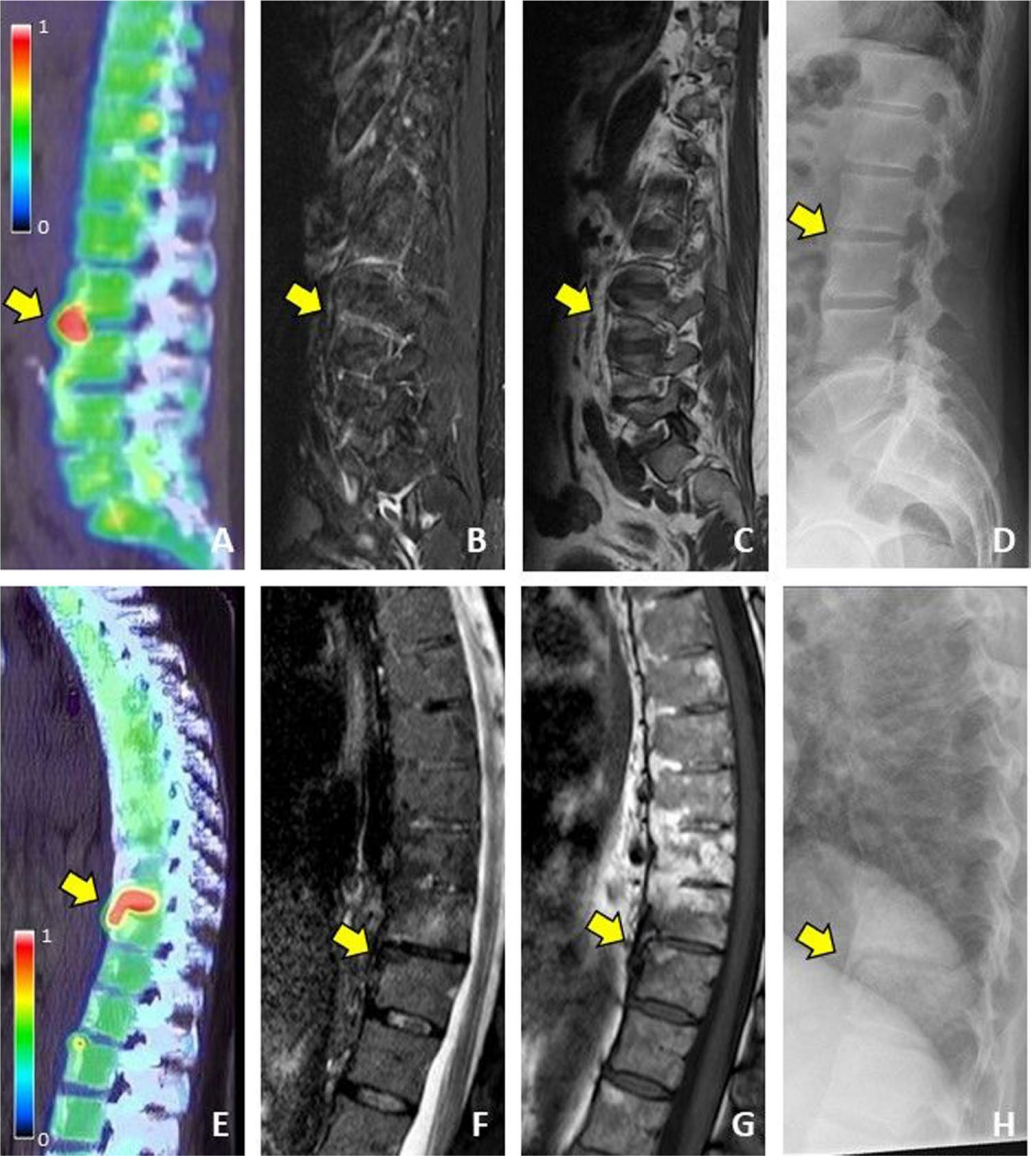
Lumbar spine of a female AS patient showing [^18^F]fluoride uptake on PET (A), no BME on MRI STIR (B^*^), no fat infiltration on MRI T1 (C^*^), and no abnormalities on CR (D), and thoracic spine of another female AS patient showing [^18^F]fluoride uptake on PET (E), minimal BME on MRI STIR (F), minimal fat infiltration on MRI T1 (G), and non-bridging syndesmophyte formation on CR (H).^*^The position of the image is caused by the lateral location of the PET lesion
